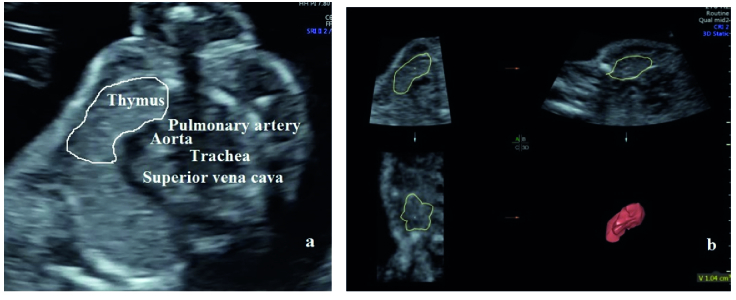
Prenatal sonographic (a) 2- and (b) 3-dimensional fetal thymus view.
